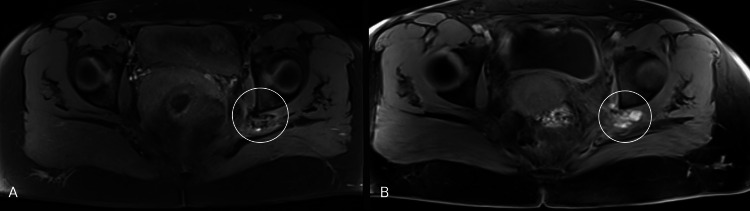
Left sciatic nerve lesion on T1 fat-suppressed MRI at six-month follow-up. (A) Initial T1 fat-suppressed image demonstrates a heterogeneous hyperintense lesion involving the left sciatic nerve, extending into the piriformis, obturator internus, and superior gemellus muscles (white circle). (B) Follow-up T1 fat-suppressed image at 6 months shows an increase in lesion size (white circle).
